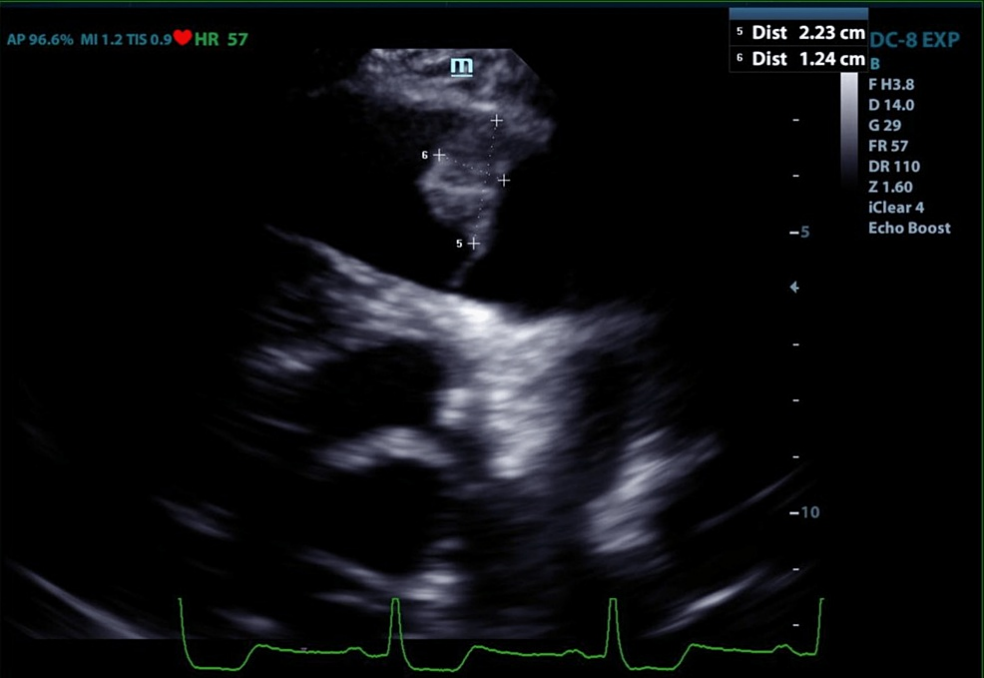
Pulmonic valve mass on follow-up transthoracic echocardiogram (parasternal short axis right ventricle outflow view)
